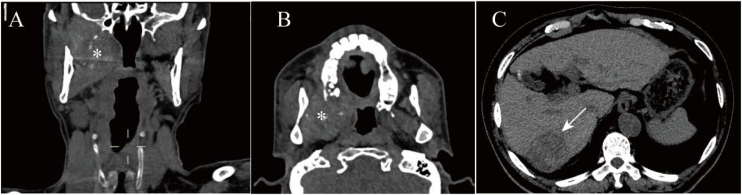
(A and B) A CT scan of the oropharynx revealed a soft tissue density mass on the right side of parapharyngeal space, involving the maxilla; (C) Abdominal CT scan showing a low-density mass with a distinct boundary in the right lobe of liver.
